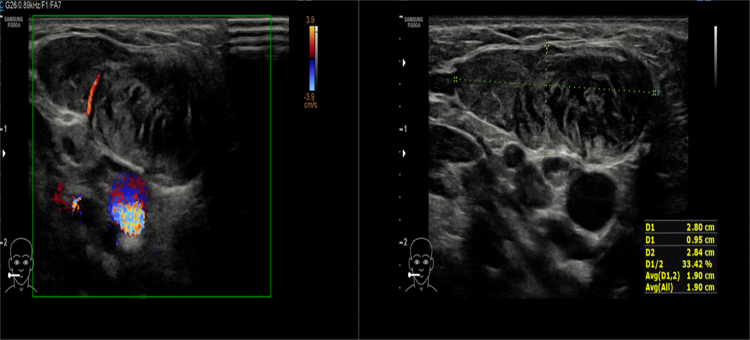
Longitudinal plane of right neck shows heterogenous hypoechoic lesion seen within the right SCM. This lesion measures 2.8 cm × 0.9 cm × 2.6 cm. No increased in colour Doppler seen within the lesion.
